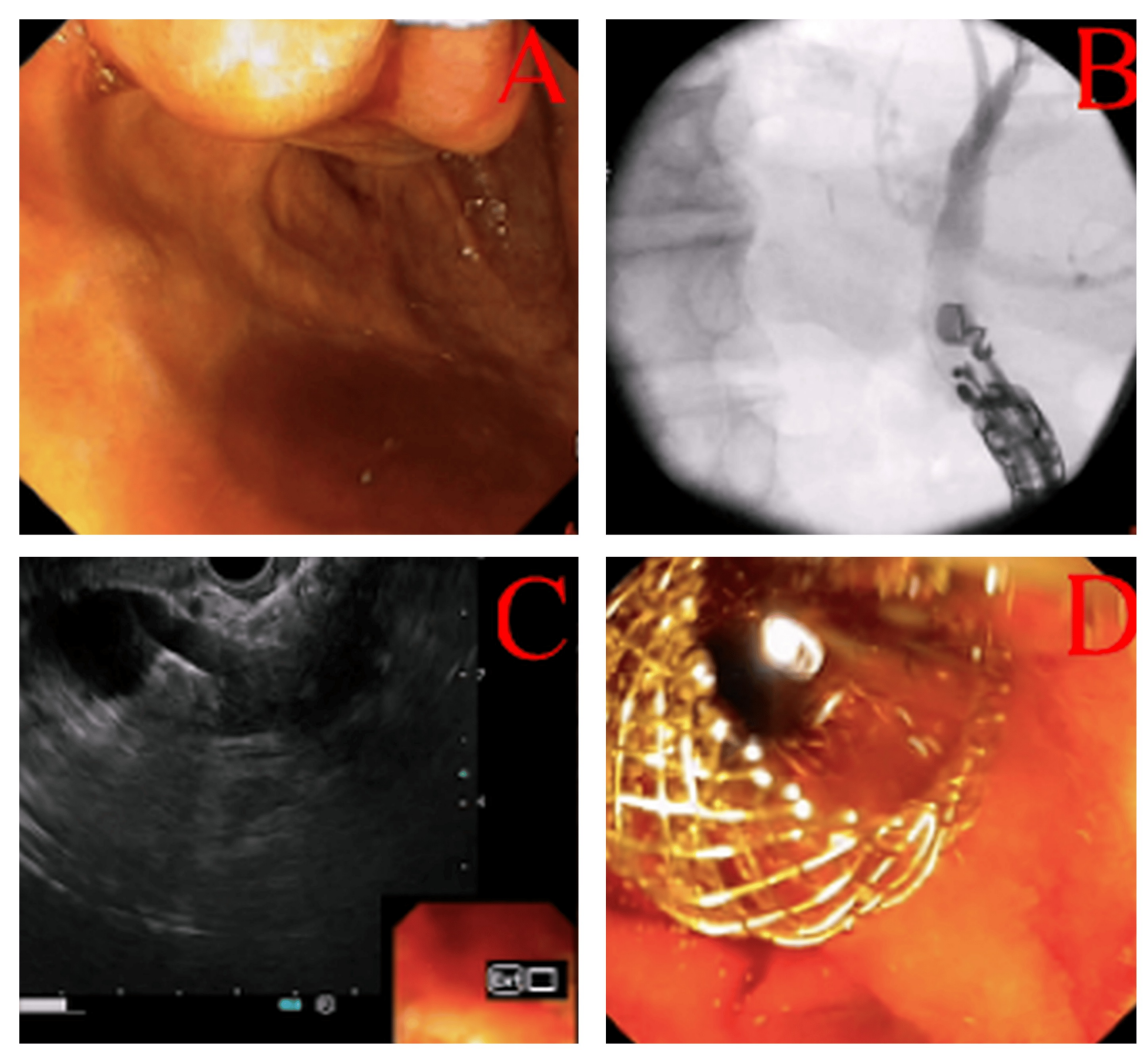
A: Cannulation trial of intradiverticular papilla at 1 o’clock, B: EUS-guided common bile duct cannulation under fluoroscopy, C: Hot-AxiosTM insertion of the common bile duct and acoustic artefacts, and D: Bile drainage inside of the choledochoduodenostomy line EUS: Endoscopic ultrasonography
